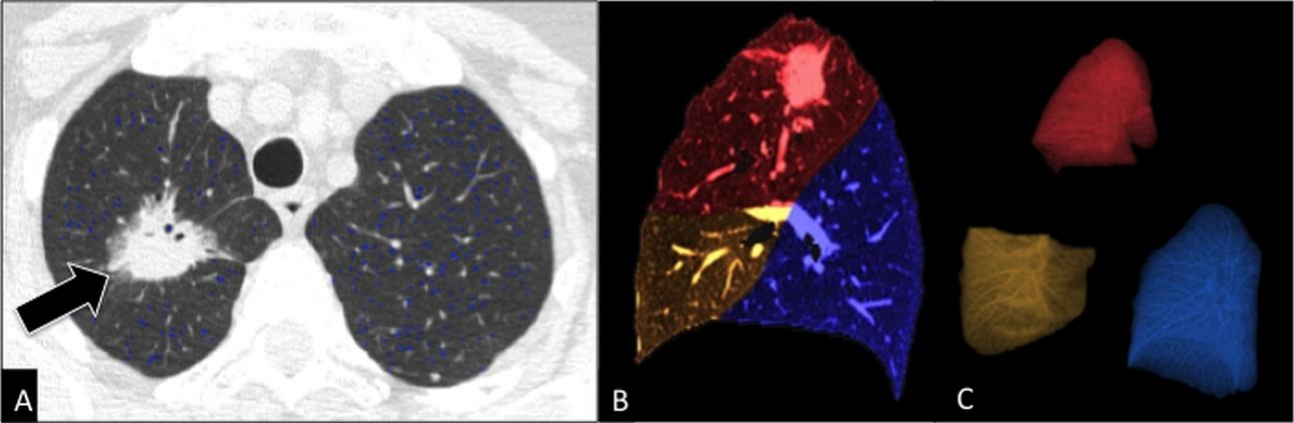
Post-processing imaging techniques: virtual lobectomy Axial CT image at lung window setting (A) shows a mass *(arrow)* in the apical segment of right upper lobe in a 73 years-old man, proved to be an invasive adenocarcinoma. Lung volume segmentation performed by using semi-automated software (Thoracic VCAR, GE Healthcare, Milwaukee, WI) allows to separate each lobe along the fissures (B, C) and to obtain the volume of emphysema (blue dots) within each lobe.
